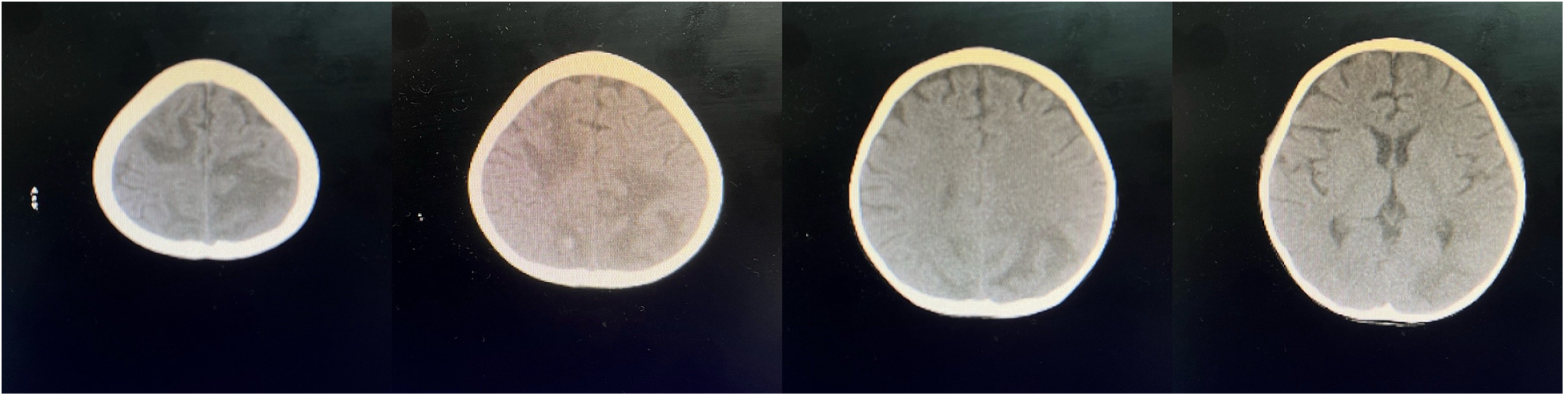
Case 1 cerebral CT demonstrated multiple bilateral frontal and parietal lobe lesions with low density, without evidence of intracranial bleeding.
